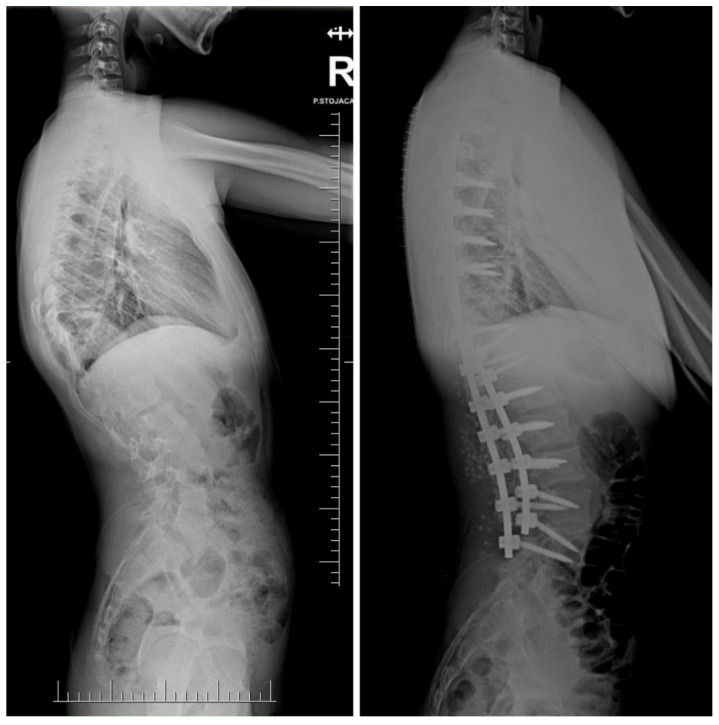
Radiographs (AP and lateral) of 17-year-old boy with congenital scoliosis and insulin-treated diabetes, who underwent P-VCR T10 (posterior-only vertebral column resection of T10).
